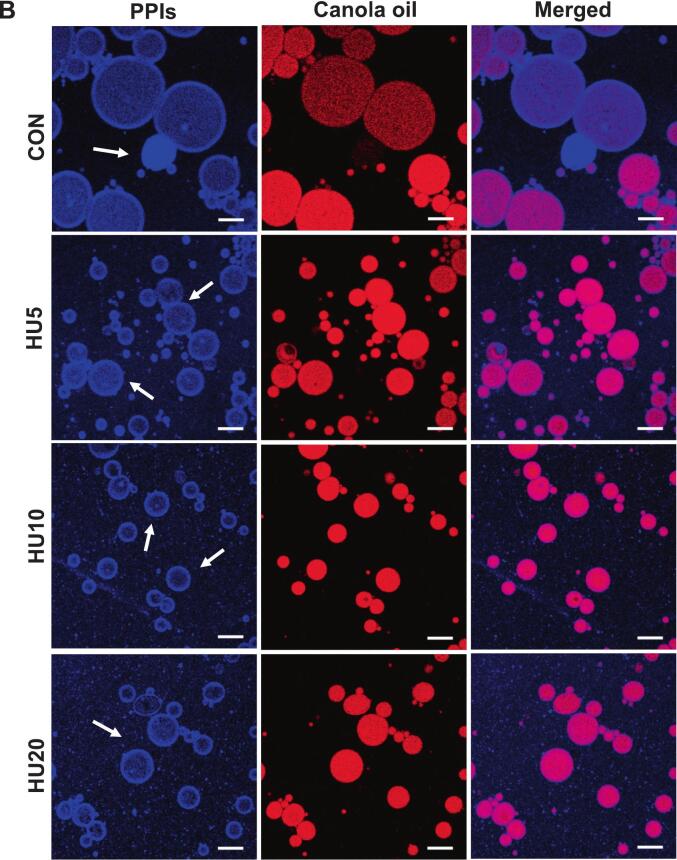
CLSM images of HIPPEs stabilized by HU-treated legume protein isolates, FBPIs (A) and PPIs (B). HIPPEs, high internal phase Pickering emulsion; FBPIs, faba bean protein isolates; PPIs, pea protein isolates; CON, untreated 4 % protein dispersions; HU, 4 % protein dispersions sequentially heated and ultrasonicated for 5, 10 and 20 min, respectively. Scale bar = 20 μm.
